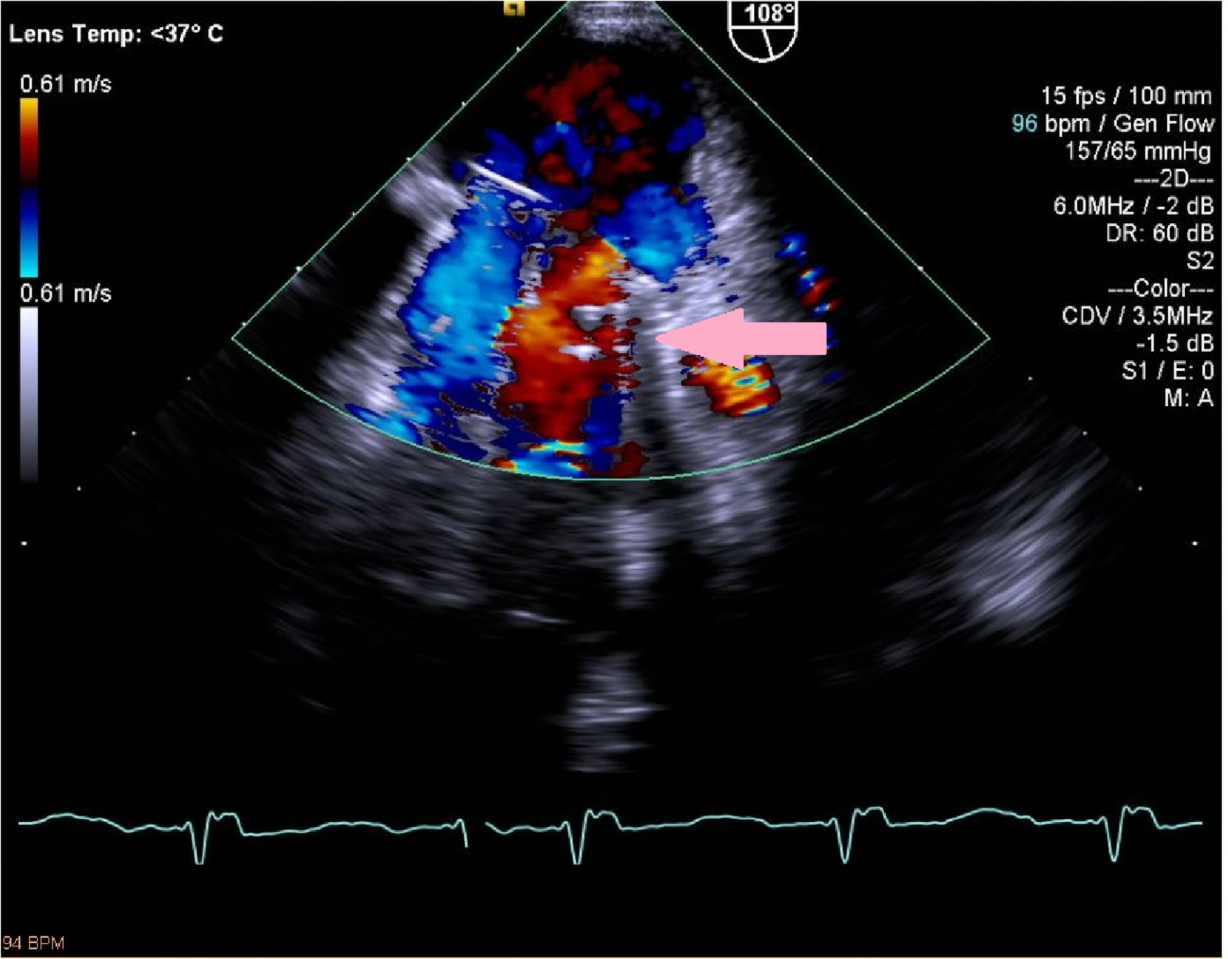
TEE image showing paravalvular leak (pink arrow) TEE, transesophageal echocardiogram
